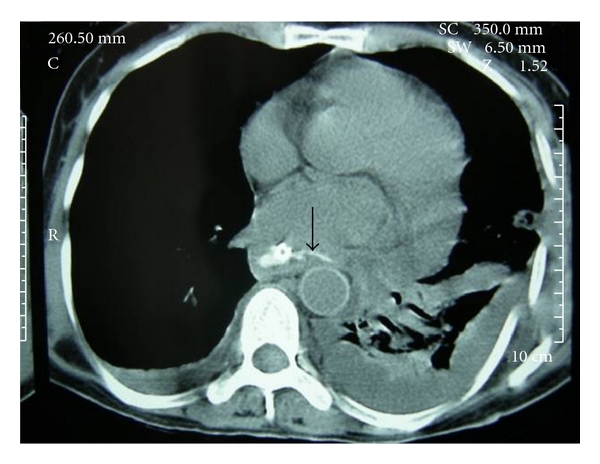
Leakage of oral contrast (arrow) from oesophagotomy repair site on CT scan.
